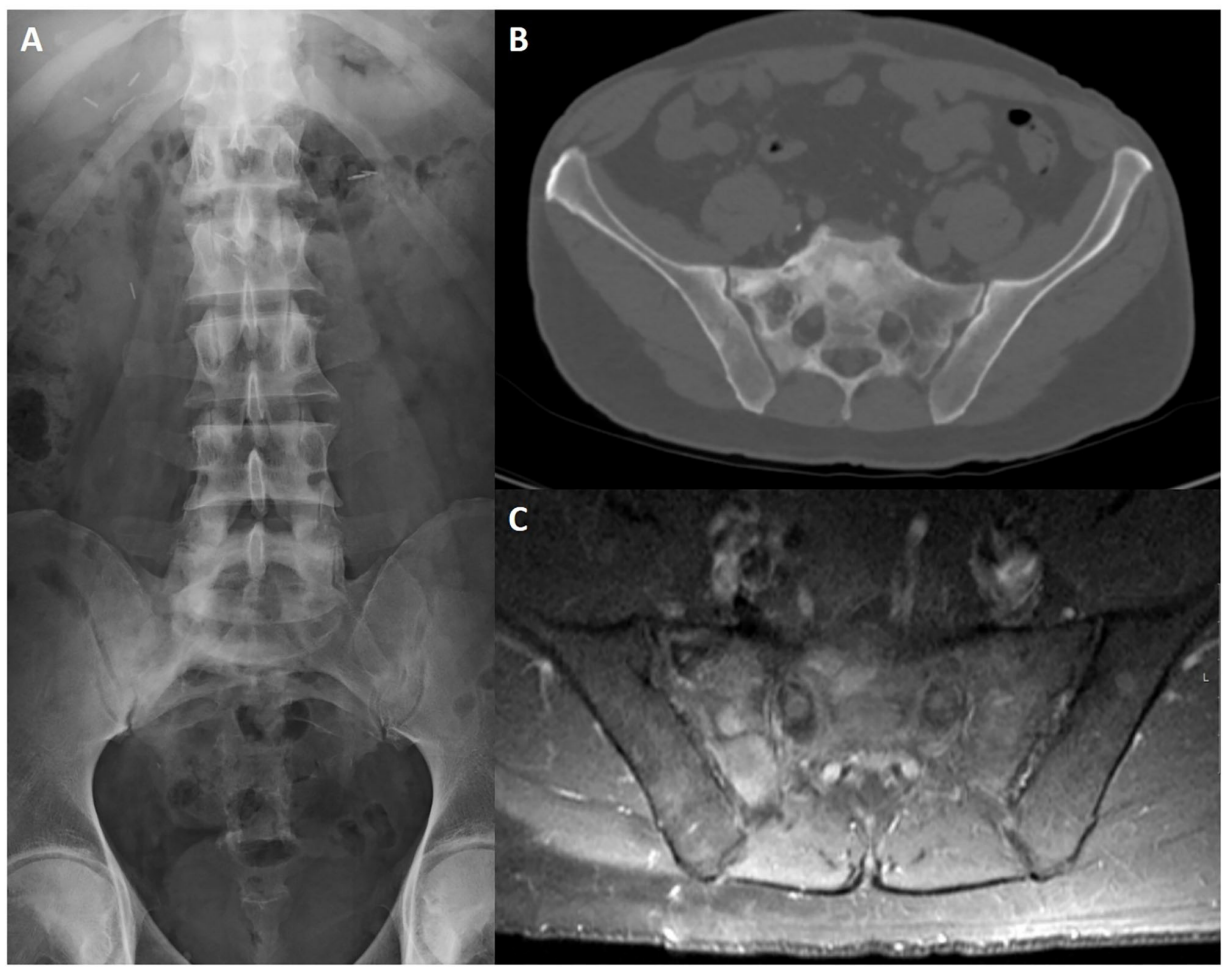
Lumbosacral radiography (A), CT axial slice (B) and post-contrast fat-saturated T1WI (C) of a 65-year-old male patient with Paget disease of the sacrum and right iliac bone. Typical findings include an expanded bone with coarsened trabecular pattern and sclerotic changes that are more evident on conventional radiography. There is increased uptake after intravenous contrast injection (C).
